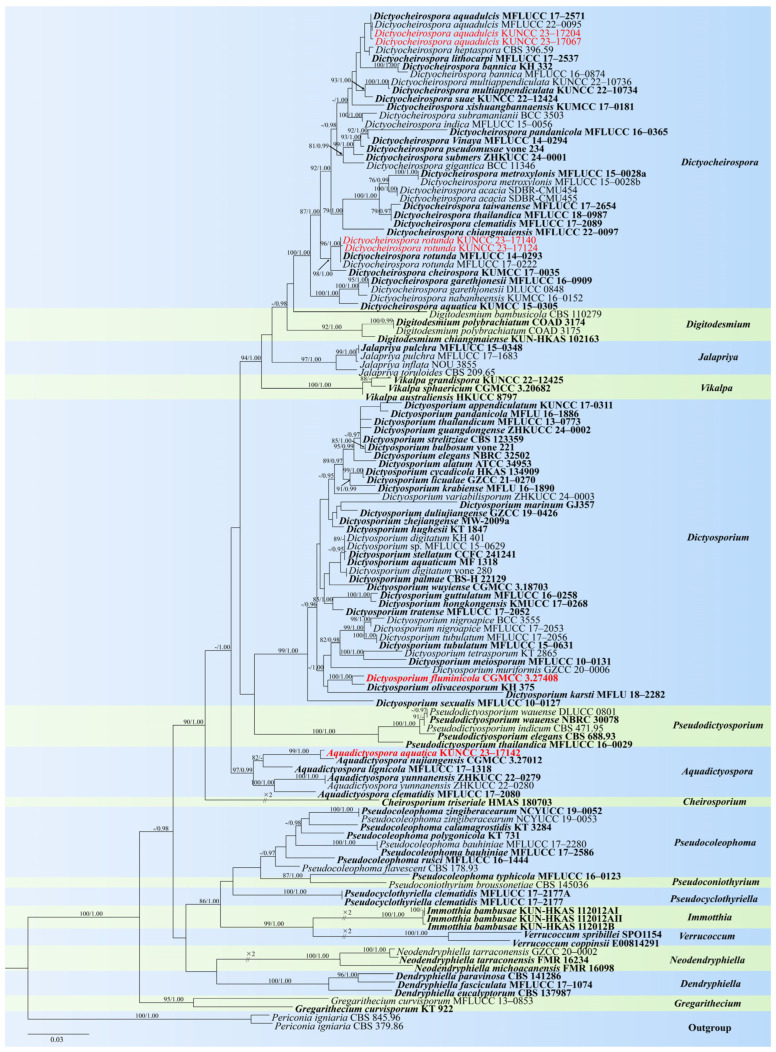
RAxML tree based on analysis of combined ITS, LSU, and *tef*1-α dataset. The combined analyses include 123 strains with 2665 characters, including gaps (ITS: 568 bp, LSU: 1255 bp, and *tef*1-α: 842 bp). The tree is rooted to *Periconia igniaria* (CBS 845.96 and CBS 379.86). The tree topology of the maximum likelihood analysis and Bayesian analysis are similar. The RAxML analysis of the combined dataset yielded a best-scoring tree with a final ML likelihood value of −22,329.430383. The matrix had 1134 distinct alignment patterns, with 33.49% undetermined characters or gaps. Estimated base frequencies were as follows: A = 0.239949, C = 0.251526, G = 0.270702, and T = 0.237823; substitution rates AC = 1.558395, AG = 3.722811, AT = 1.999671, CG = 0.838522, CT = 8.185164, and GT = 1.000000; and gamma distribution shape parameter α = 0.224379. Bootstrap values for maximum likelihood (ML) of ≥75% and clade credibility values of ≥0.95 from Bayesian-inference analysis are labeled on the nodes. The ex-type strains are in bold. The newly obtained sequences are indicated in red.
